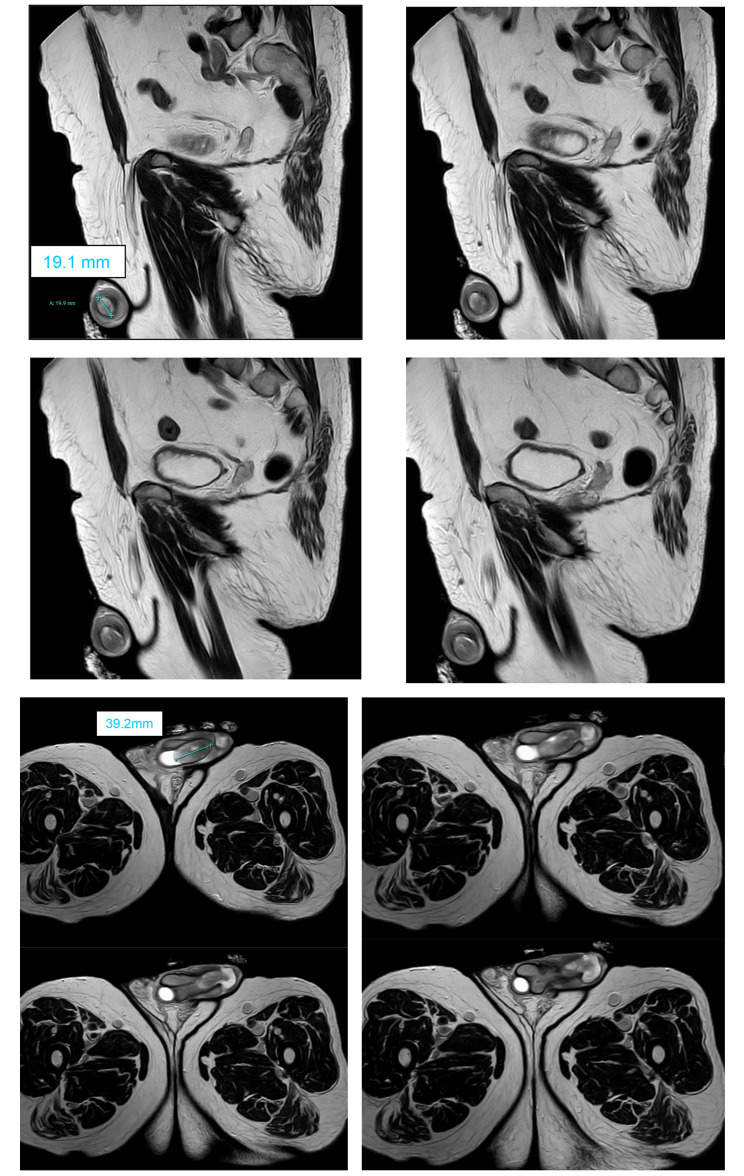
MRI of the tumor formation with size indication
